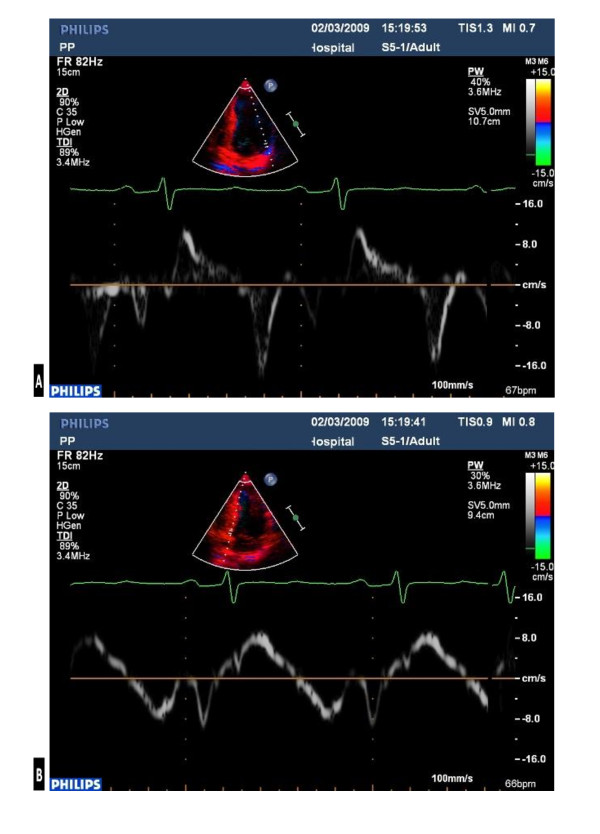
Tissue Doppler analysis of longitudinal function; with an E' of 16 cm/s in the lateral LV wall (a) and 11 cm/s in the septal LV wall (b).
